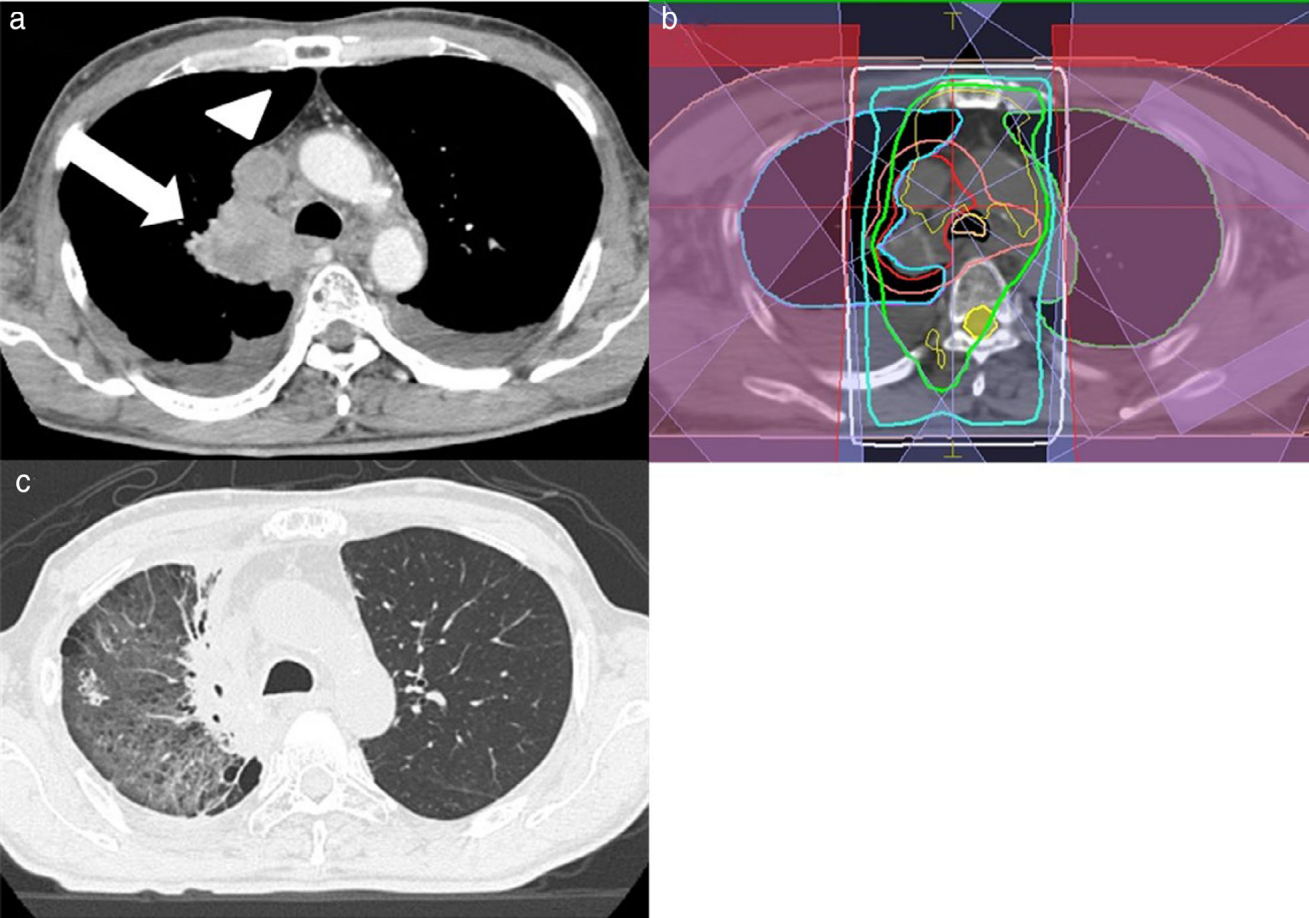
(a) Computed tomography (CT) shows primary lung cancer of the right hilum (arrow) with superior vena cava syndrome (arrowhead) in a man in his 60s. (b) He received three‐dimensional radiotherapy; isodose lines of 100% (yellow), 95% (light green), 85% (light blue), 50% (white), and the planned target volume (pink) are shown. (c) After 12 courses of an anti‐PD‐1 antibody (24 months after thoracic radiation therapy), CT showed new ground‐glass opacity in the irradiated area, which had also spread to the low‐dose area and outside the irradiated area. He was diagnosed with organizing pneumonitis.
